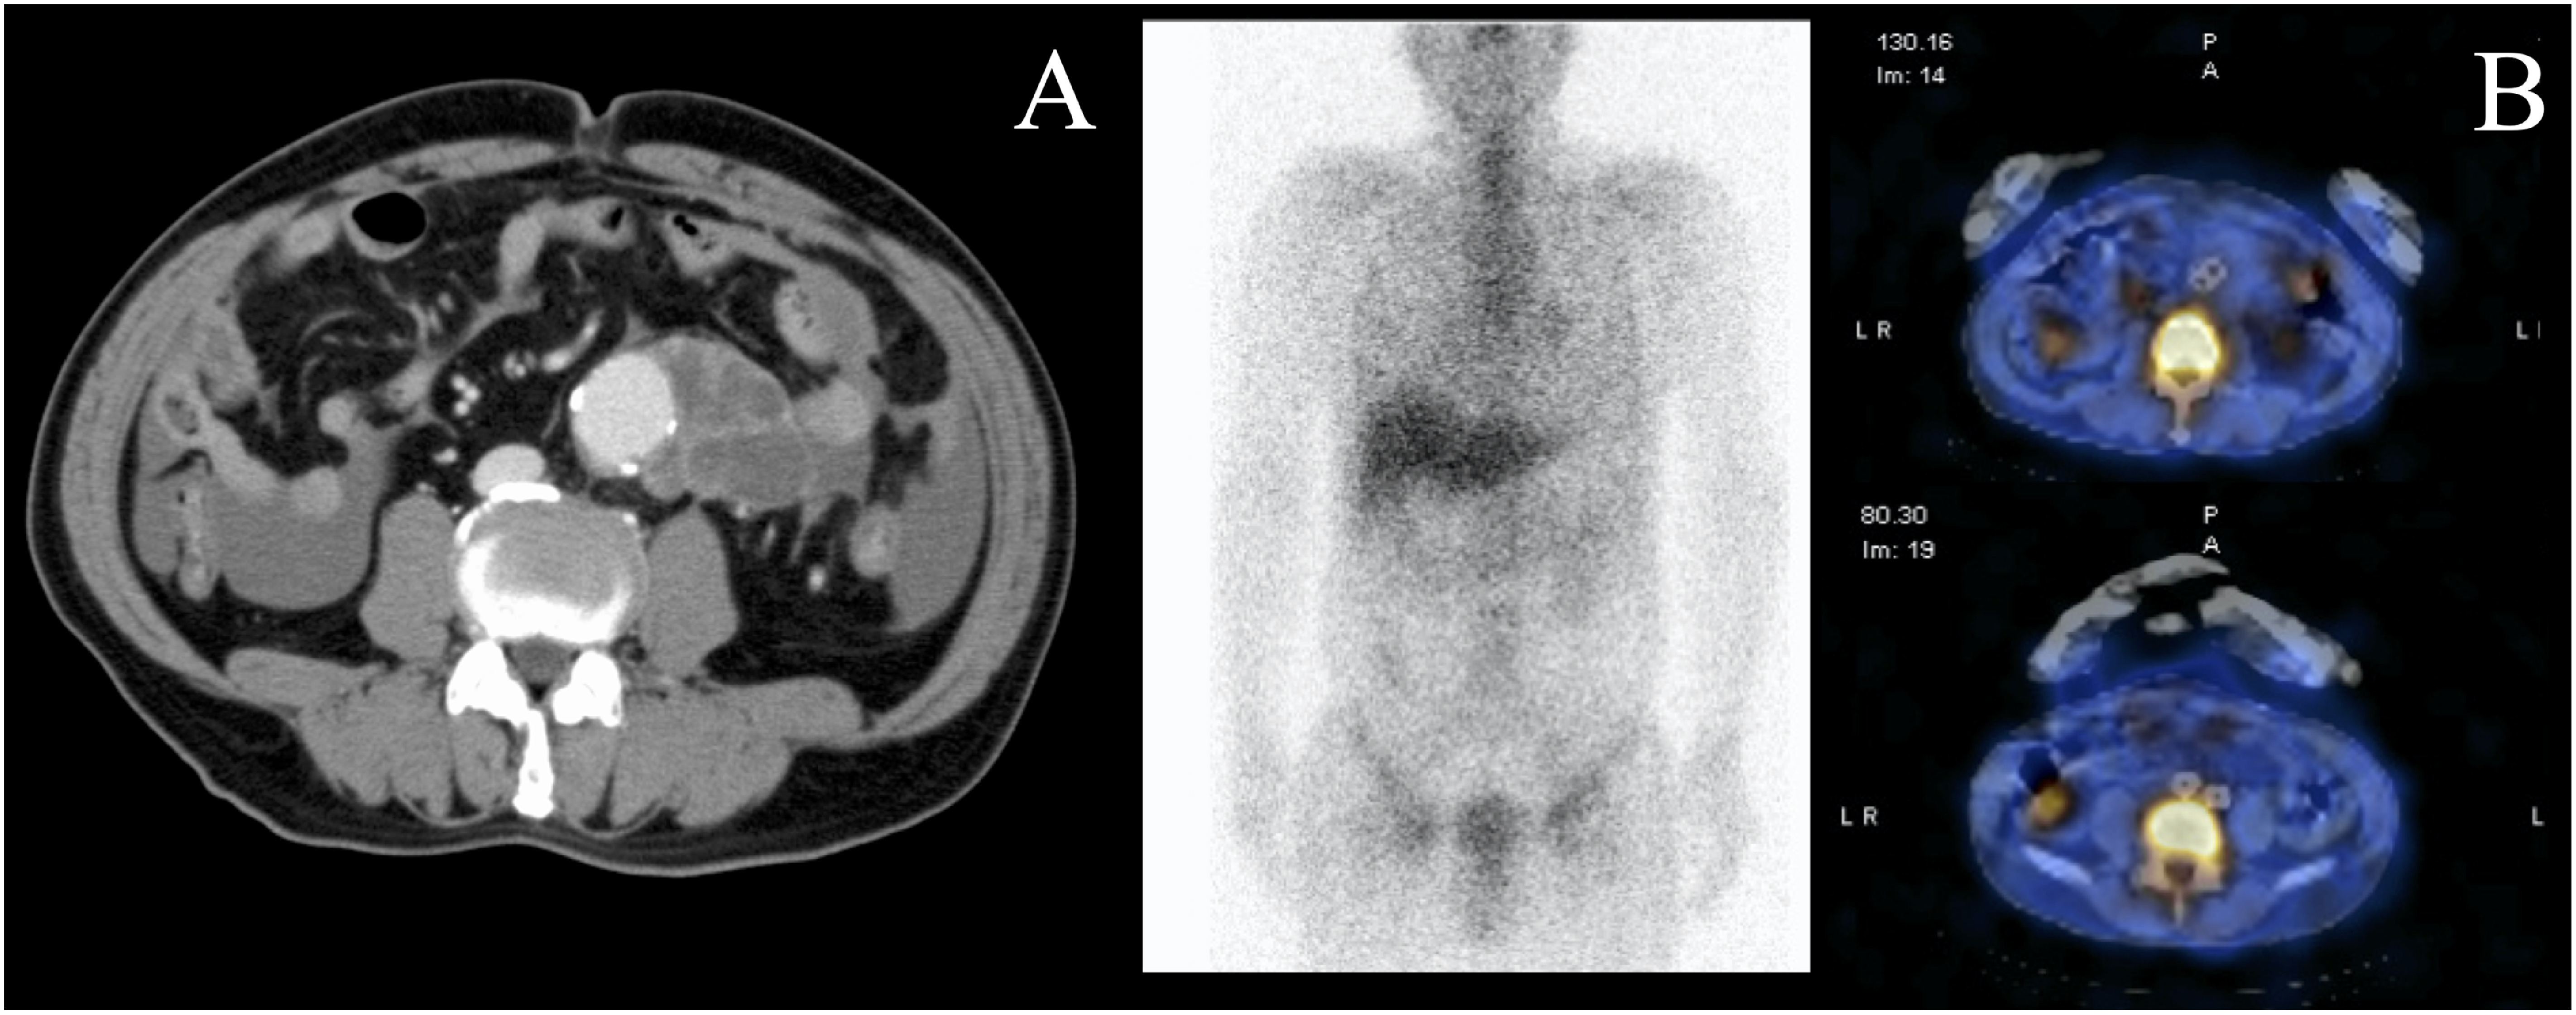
Fig. 1 An enhanced computed tomography (CT) and Ga-67-citrate scintigraphy before endovascular aortic repair. (A) CT showed an abdominal aortic aneurysm (AAA) surrounded by diverse cystic structures up to 52 mm in diameter. (B) A Ga-67-citrate scintigraphy showed no significant uptake of Ga-67-citrate around the AAA and stent-graft.
